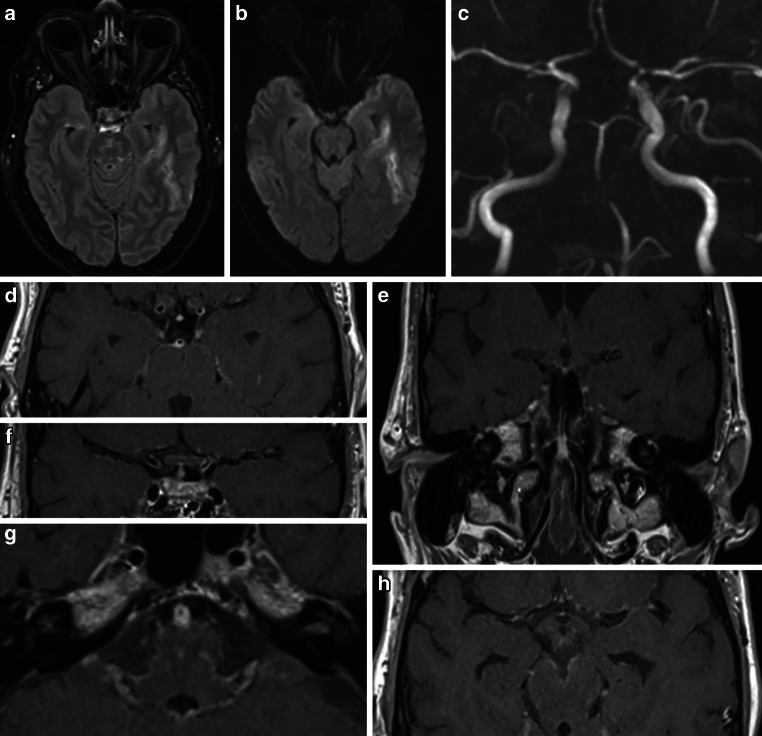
MRI of a 57-year-old patient in the early acute phase of neuroborreliosis-induced vasculitis on day 1. FLAIR (axial view; a) and DWI image (axial view; b) demonstrating new ischemic area in the left temporal lobe. MIP of the TOF angiography (c) confirming previously seen intracranial stenoses (distal left ICA, left ACA/A1, left MCA—M1 and superior trunk, bilateral vertebral artery/V4, proximal basilar artery, bilateral PCA/P2). Fat-saturated post-contrast T1-weighted images (axial views—d, f, g, h; coronal view—e) showing mural thickening and contrast enhancement in stenotic vessel segments, and basal leptomeningeal contrast enhancement
